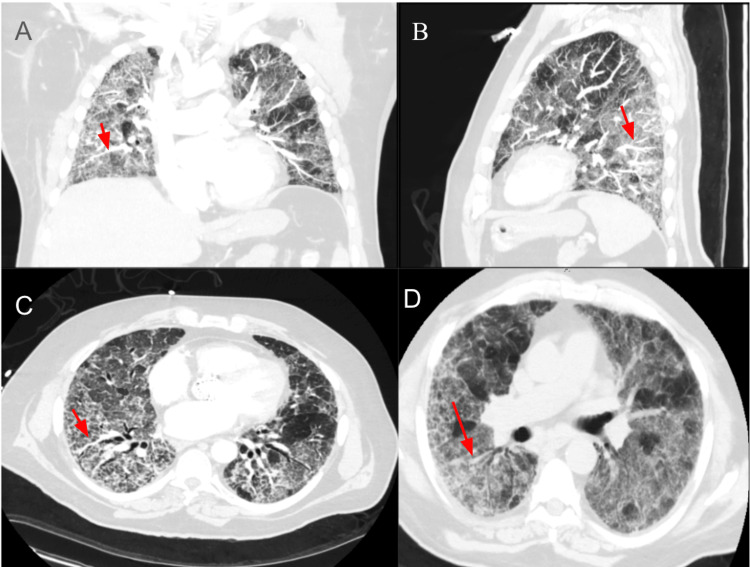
Computed tomography angiography (CTA) of the chest demonstrating diffuse interstitial lung disease (panels A, B, C, D), characterized by bilateral ground-glass opacities (red arrows in panels B & D) and interlobular septal thickening (red arrows in panels A & C). No evidence of honeycombing is observed.
